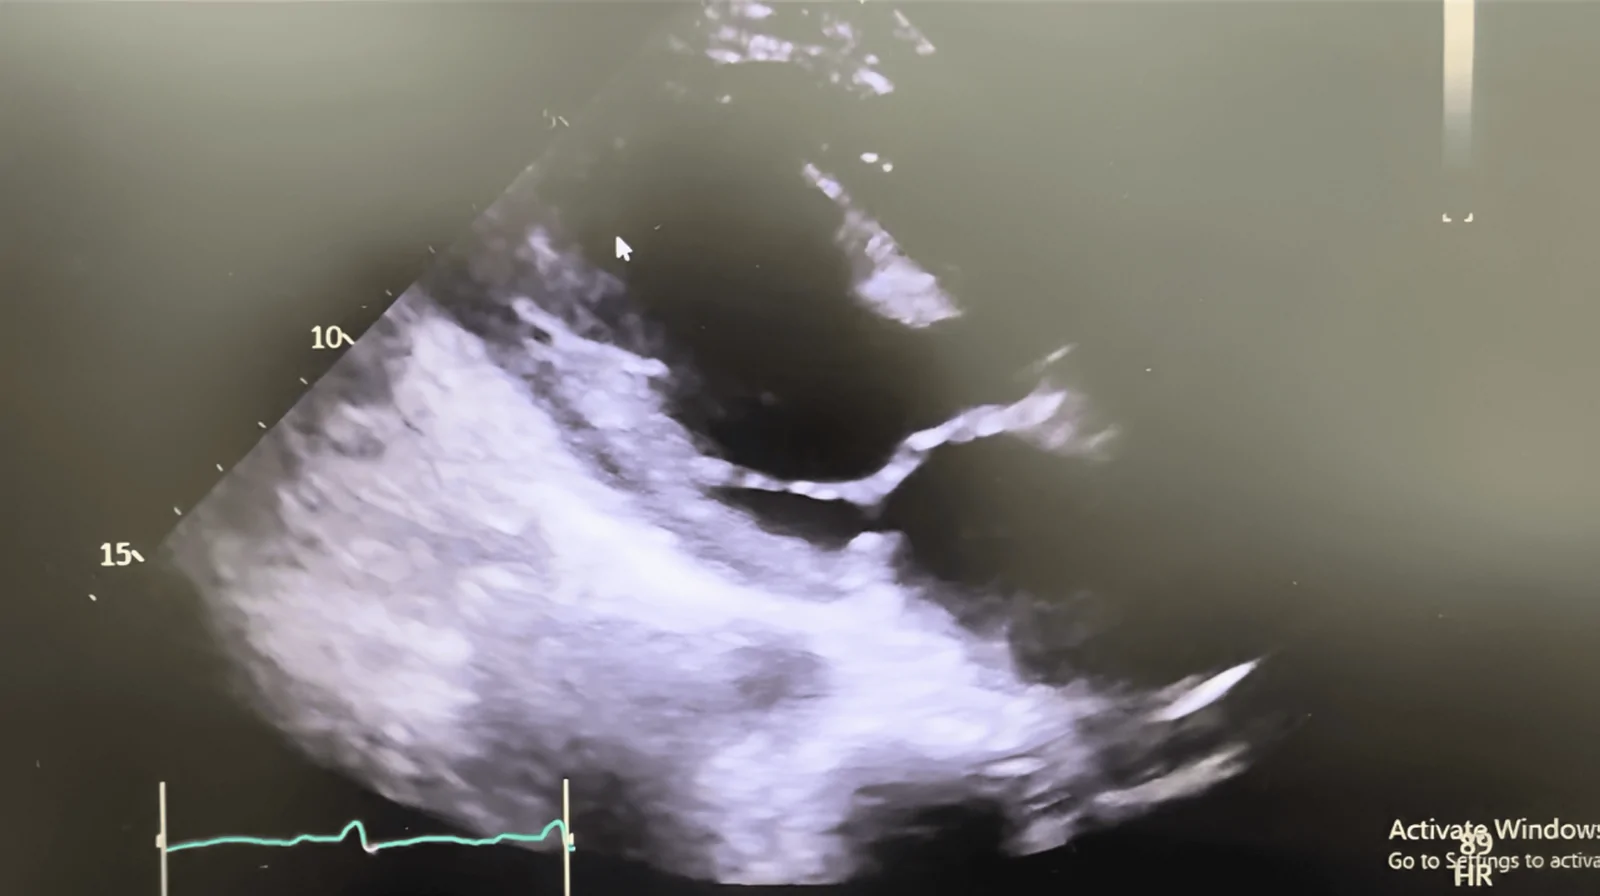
Follow-up transthoracic echocardiographic image obtained in August 2025 demonstrating severe left ventricular systolic dysfunction without a visible residual left ventricular apical thrombus. This image represents the non-contrast follow-up view; the clinical echocardiography report also documented no residual thrombus on contrast-enhanced imaging.
